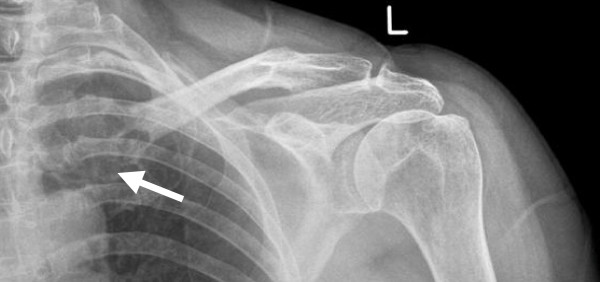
Plain radiograph of the left shoulder taken 4 months later. The radiograph demonstrates a large lytic lesion (arrow) over the medial aspect of the clavicle.
